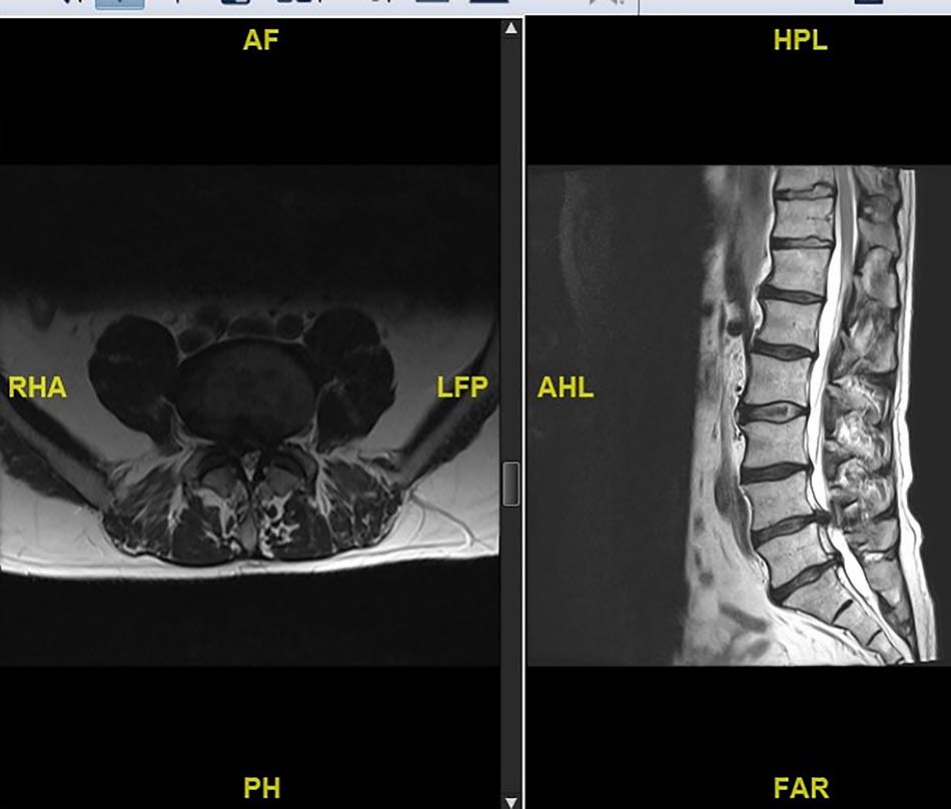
Preoperative MRI scan showing L4/5 disc prolapse.
